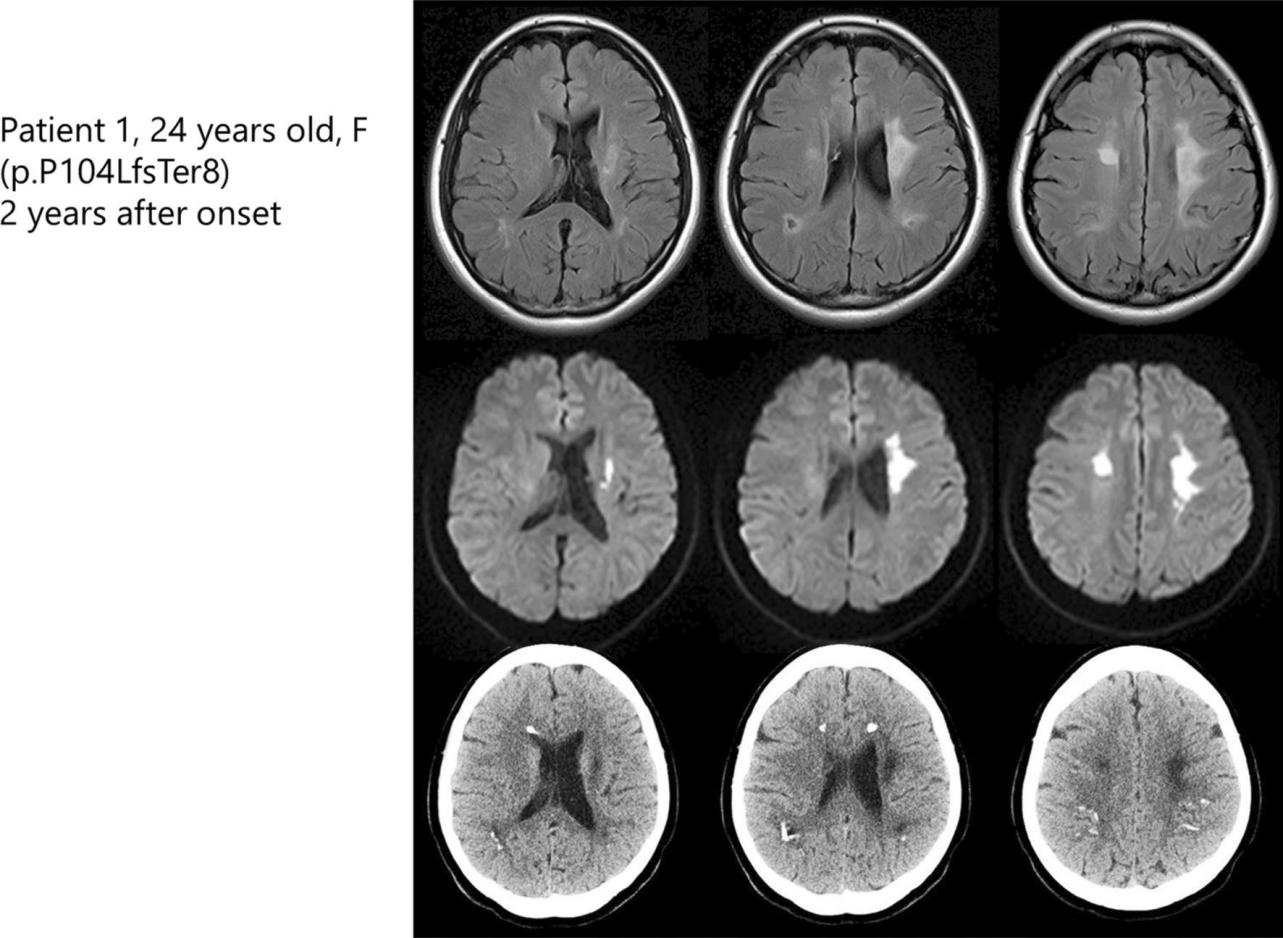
Brain MRI and CT findings of ALSP patient with the frameshift mutation in exon 4. Characteristic hyperintensities in the white matter are observed in fluid-attenuated inversion recovery MR images (upper panels). Diffusion-weighted MR images revealed high-intensity lesions with left-side predominance (middle panels). CT scan revealed small spotty calcifications in the frontal and parietal white matter (lower panels)
